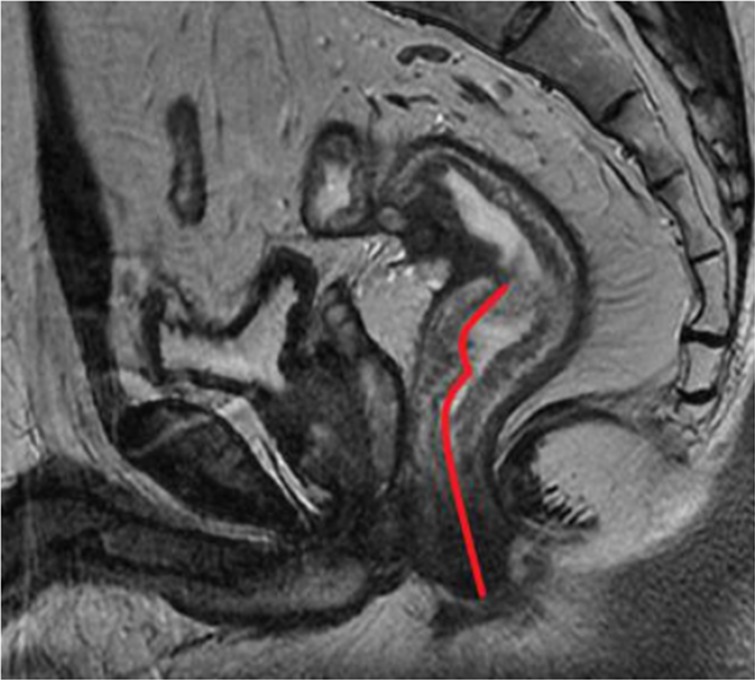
The red curved line from from the inferior part of the tumor to the anal verge indicates the measurement of distance from tumor to anal verge (DTA) on sagittal T2-weighted imaging
